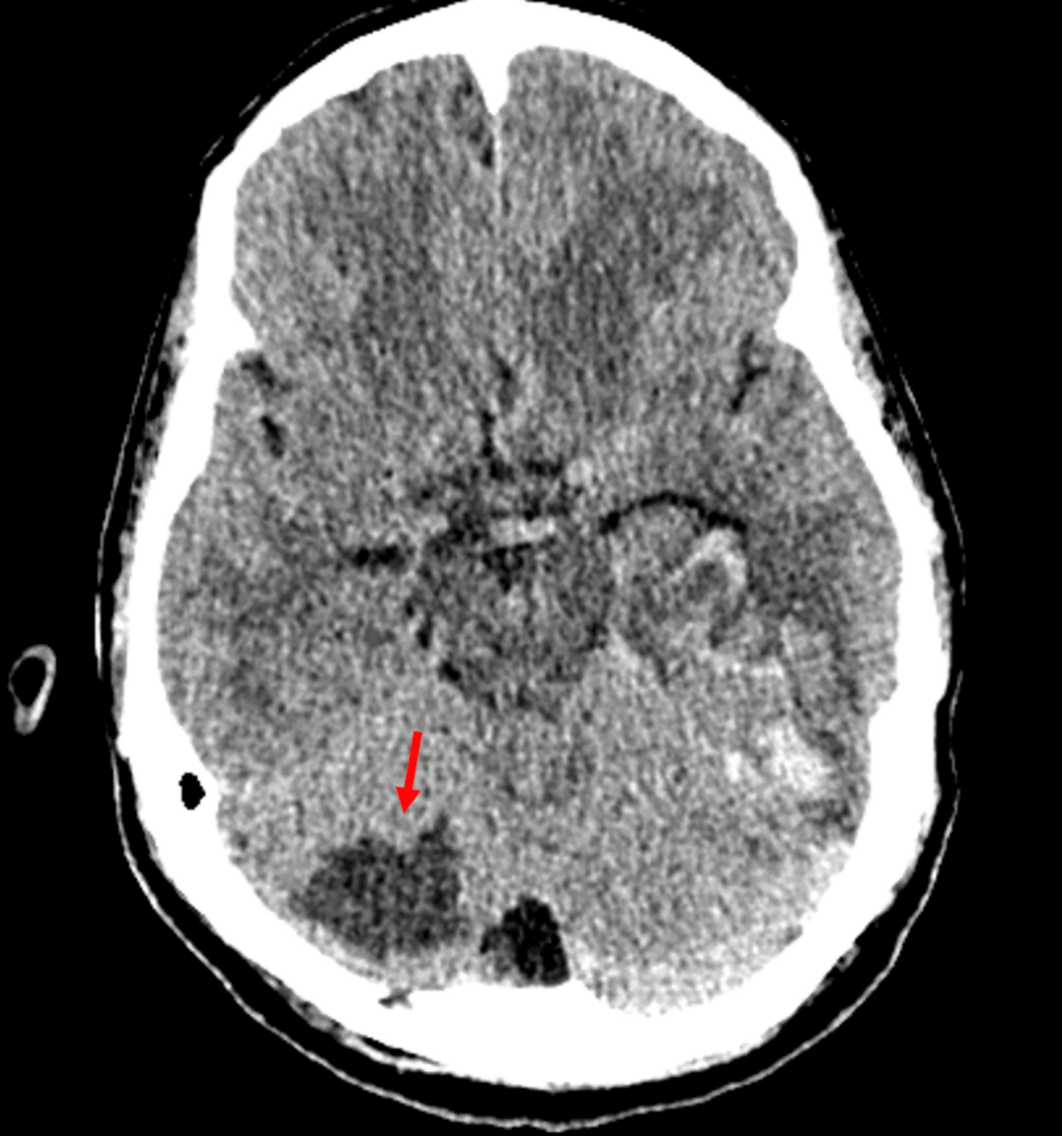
Non-contrast brain CT. The red arrow is pointing to the hypodense lesion.
